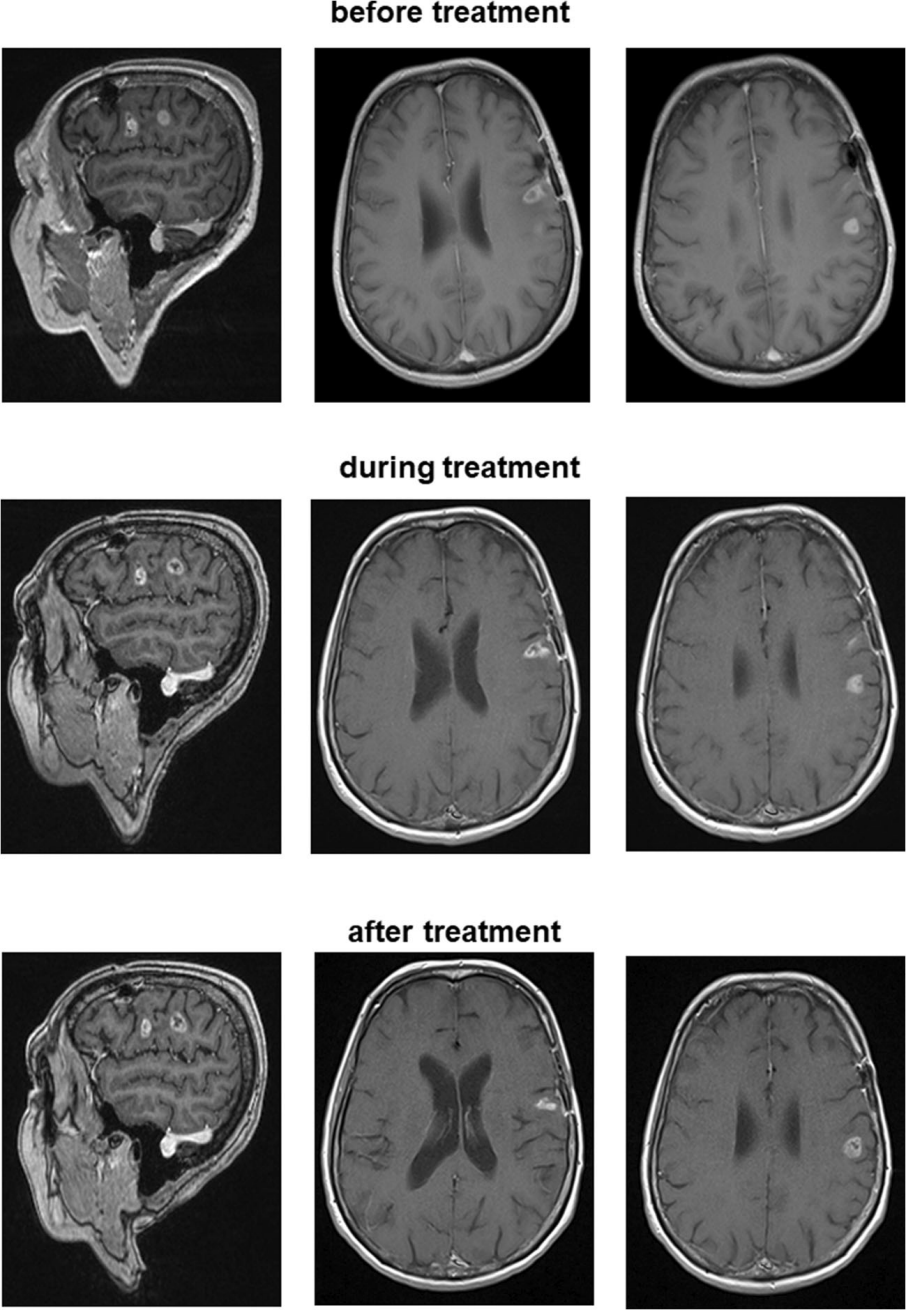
Computed tomography (CT) scans and magnetic resonance images (MRI) before, during and after the temozolomide treatment of the glioblastoma patient
